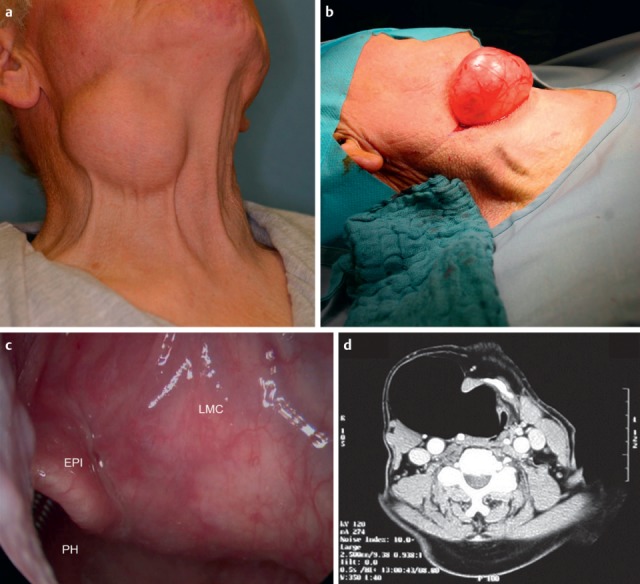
73-year-old patient with advanced right-sided internal and external laryngocele. Increasing dysphagia and muffled speech: a – clearly prominent mass at the right side of the neck; b – intraoperative situation; c – preoperative endoscopic image (LC – laryngocele, EPI – epiglottis, PH – posterior pharyngeal wall); d – horizontal CT scan of the neck with advanced right-sided laryngocele.
